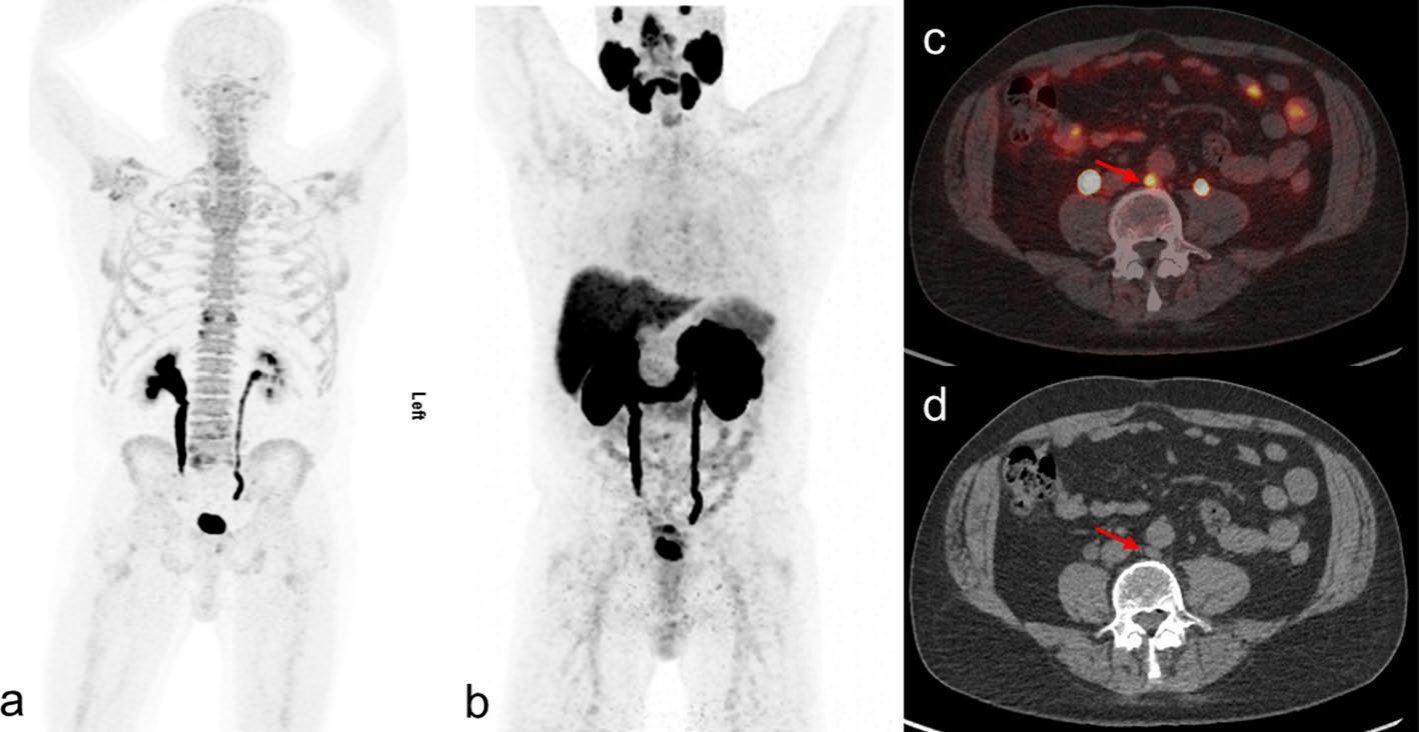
A 75-year-old 4 + 3 PCa patient treated with radiation and adjuvant hormonal therapy referred for PET imaging due to rising PSA (PSA was 42 ng/mL at time of scan). Maximum intensity projection images of both fluoride PET (a) and PSMA PET (b) showed negative bone lesions. However, para-aortic lymph node (red arrow) showed positive uptake on PSMA PET (c), which also correlated with CT (d)
